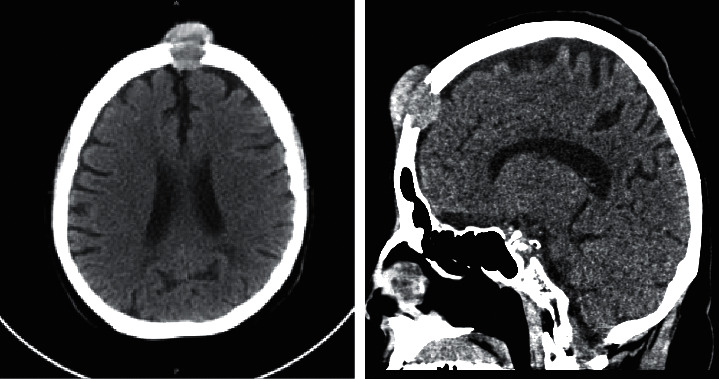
Cross-sectional and sagittal images of CT head (with and without contrast) demonstrating a 3.1 cm × 3 cm × 3 cm enhancing soft tissue mass eroding through the frontal calvarium and extending into the anterior soft tissues of the scalp in the epidural space overlying the left frontal lobe.
